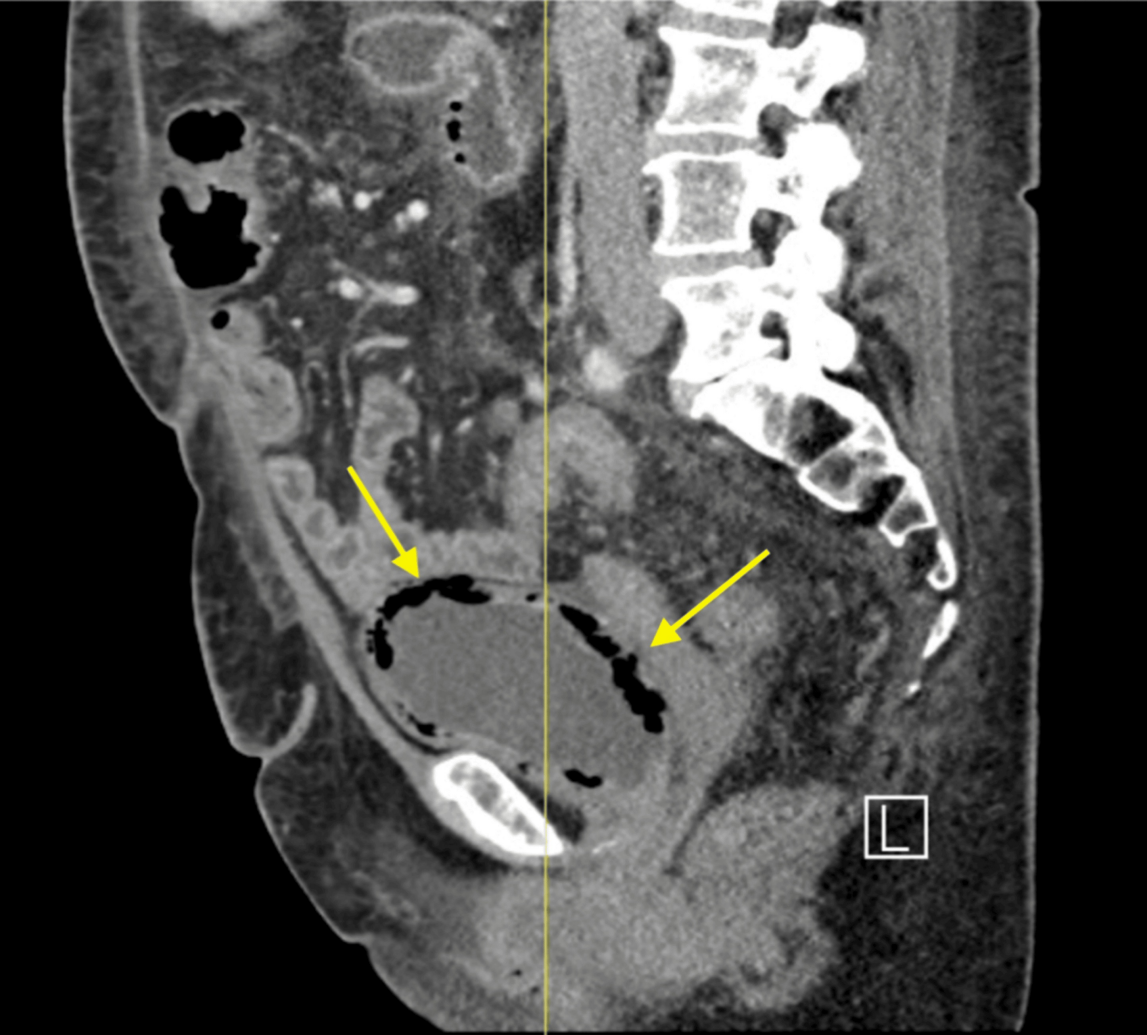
CT image displaying pneumatosis of the bladder wall consistent emphysematous cystitis in Case 3 CT: computed tomography
